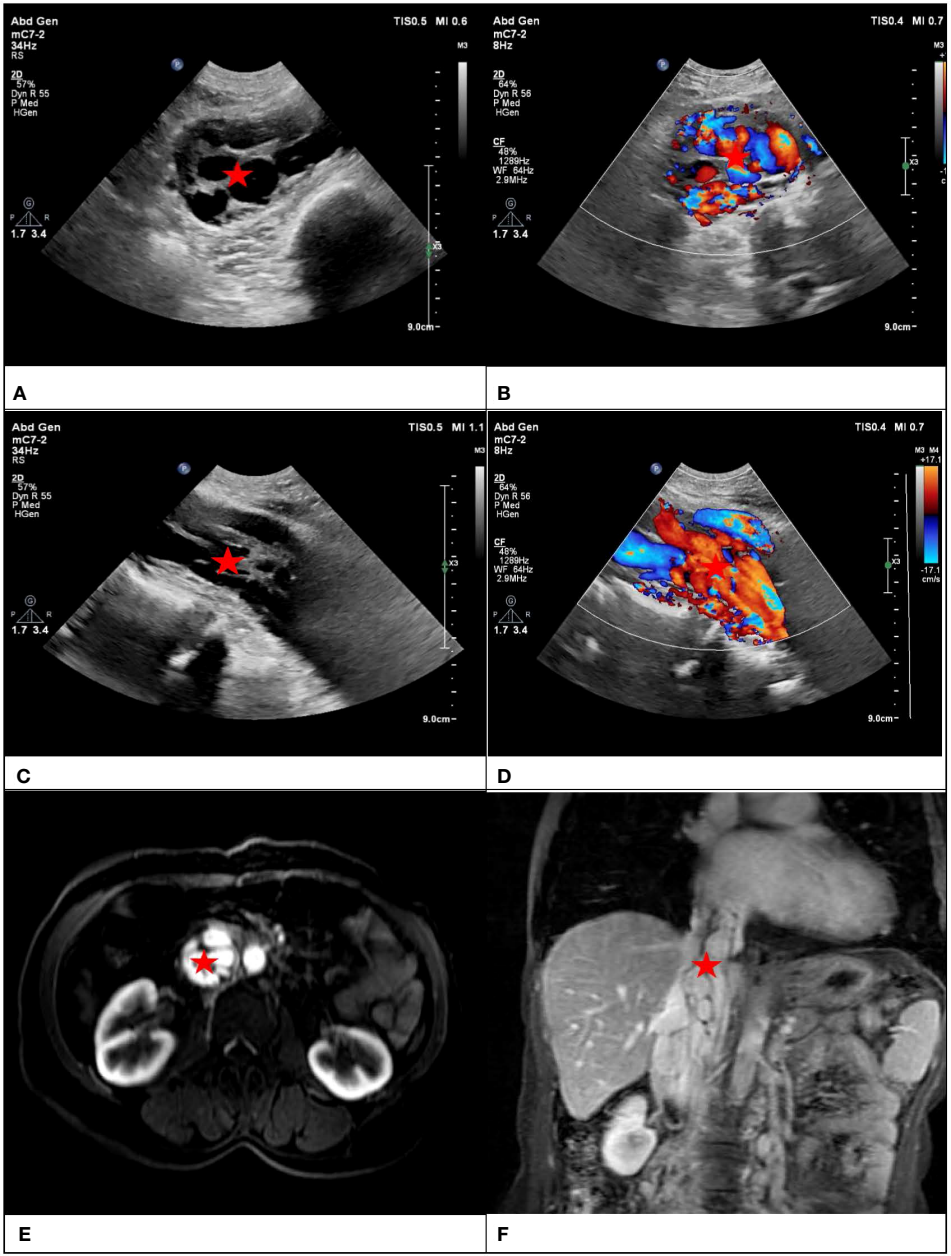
Transverse section of IVL showed the “sieve hole sign” in gray scale mode and color Doppler mode in figure (A, B) respectively (red star). Longitudinal section of IVL showed the “multi-track sign” in gray scale mode and color Doppler mode in figure (C, D) respectively (red star). Figure (E) showed transverse section of IVL with “sieve hole sign” and figure (F) showed longitudinal section with “multi-track sign” in enhanced MRI (red star).
